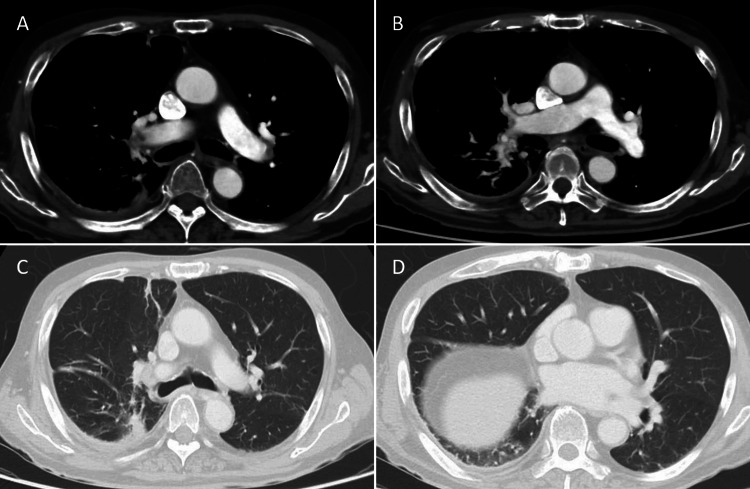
Follow-up CT images prior to patient transfer Follow-up CT scans performed prior to transfer of the patient show significant radiologic improvement following 32 days of saline irrigation. A and B demonstrate nearly complete resolution of the previously noted pulmonary embolism. C and D show marked improvement in pleural space condition, with no new fluid collections or evidence of pneumonia, correlating with cessation of purulent drainage and air leak. These results allowed for the successful discontinuation of oxygen and antibiotics, chest tube removal, and eventual patient transfer to a rehabilitation facility in stable condition. CT, computed tomography
